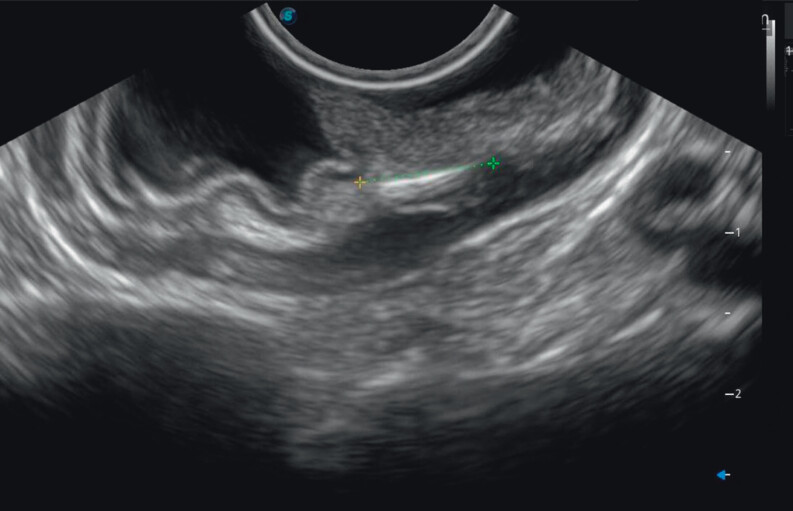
Endoscopic ultrasonogram identifying the location and depth of the fish bone within the muscularis propria.
